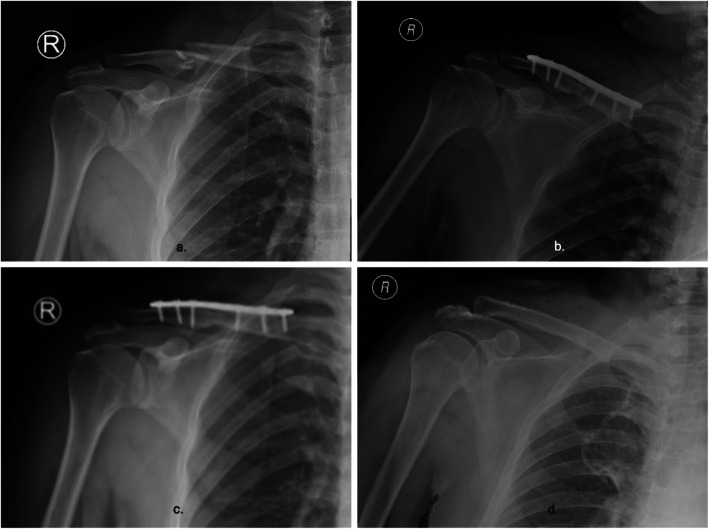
Imaging follow-up of Nice knot as an auxiliary reduction technique in the treatment of clavicle fracture. a The preoperative X-ray showed a displaced comminuted fracture of the clavicle. b On the first day after operation, routine X-ray examination showed that the alignment of the fracture was good. Three months after (c), the X-ray showed that the fracture healed well. Twelve months after (d), the internal fixation device was removed and X-ray examination showed that there was no fracture
